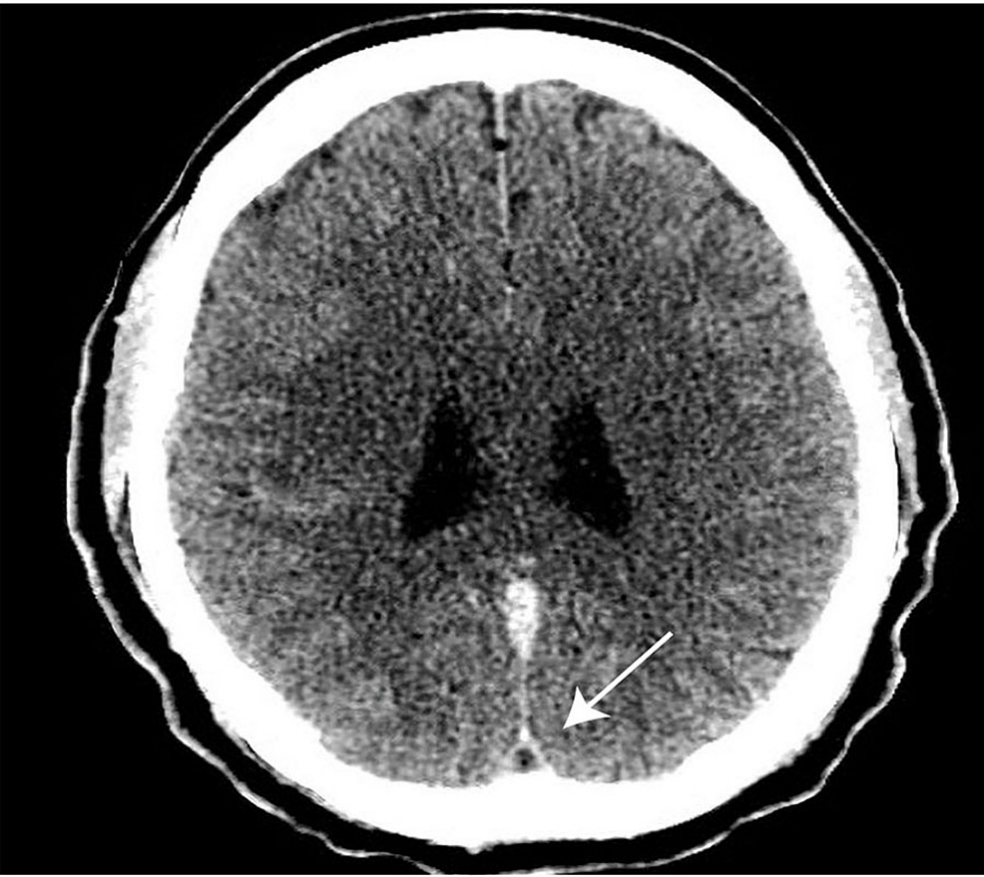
CT angiogram (axial sequence) showing "empty delta" sign.
